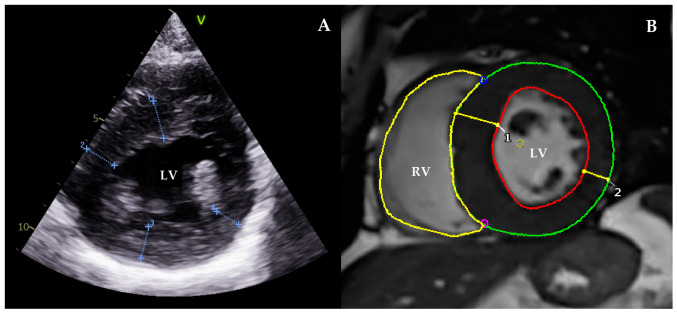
Short-axis maximal MWT measurement by TTE (A) and CMR (B). In the TTE image, 1 and 2 indicate the interventricular septum and 3 and 4 indicate the posterior wall. In the CMR image, 1 indicates the interventricular septum and 2 indicates the posterior wall. CMR: Cardiac magnetic resonance; LV: Left ventricle; RV: Right ventricle; MWT: Myocardial wall thickness; TTE: Transthoracic echocardiography.
